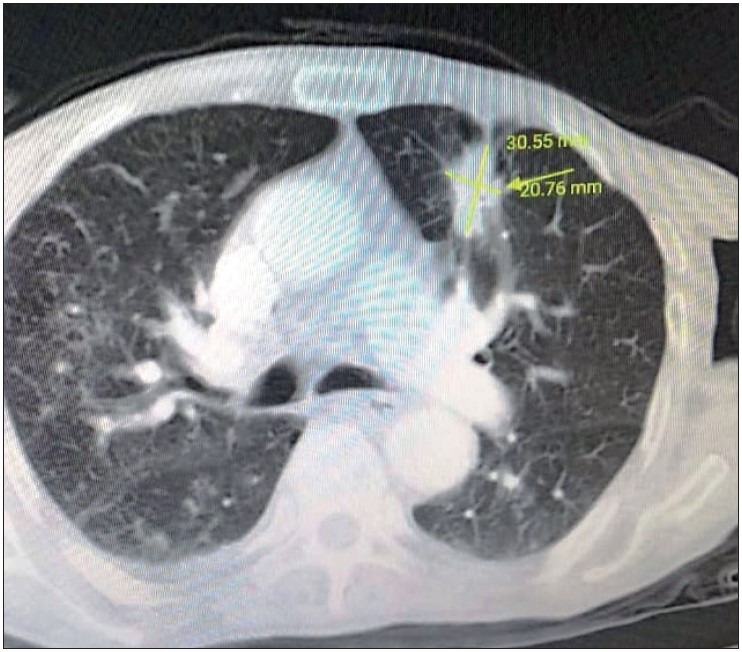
CT revealed a mass of 3x2 cm on the superior lobe of the left lung (yellow arrow).
